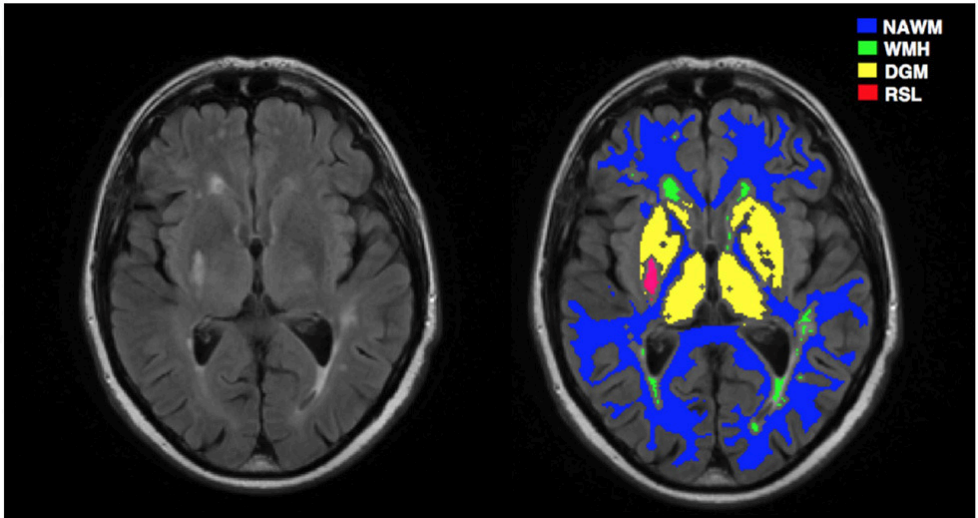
A representative MRI image and corresponding tissue segmentations. FLAIR image (left) and tissue masks superimposed on FLAIR image (right). NAWM, normal-appearing white matter; WMH, white matter hyperintensities; DGM, deep gray matter; RSL, recent stroke lesion.
